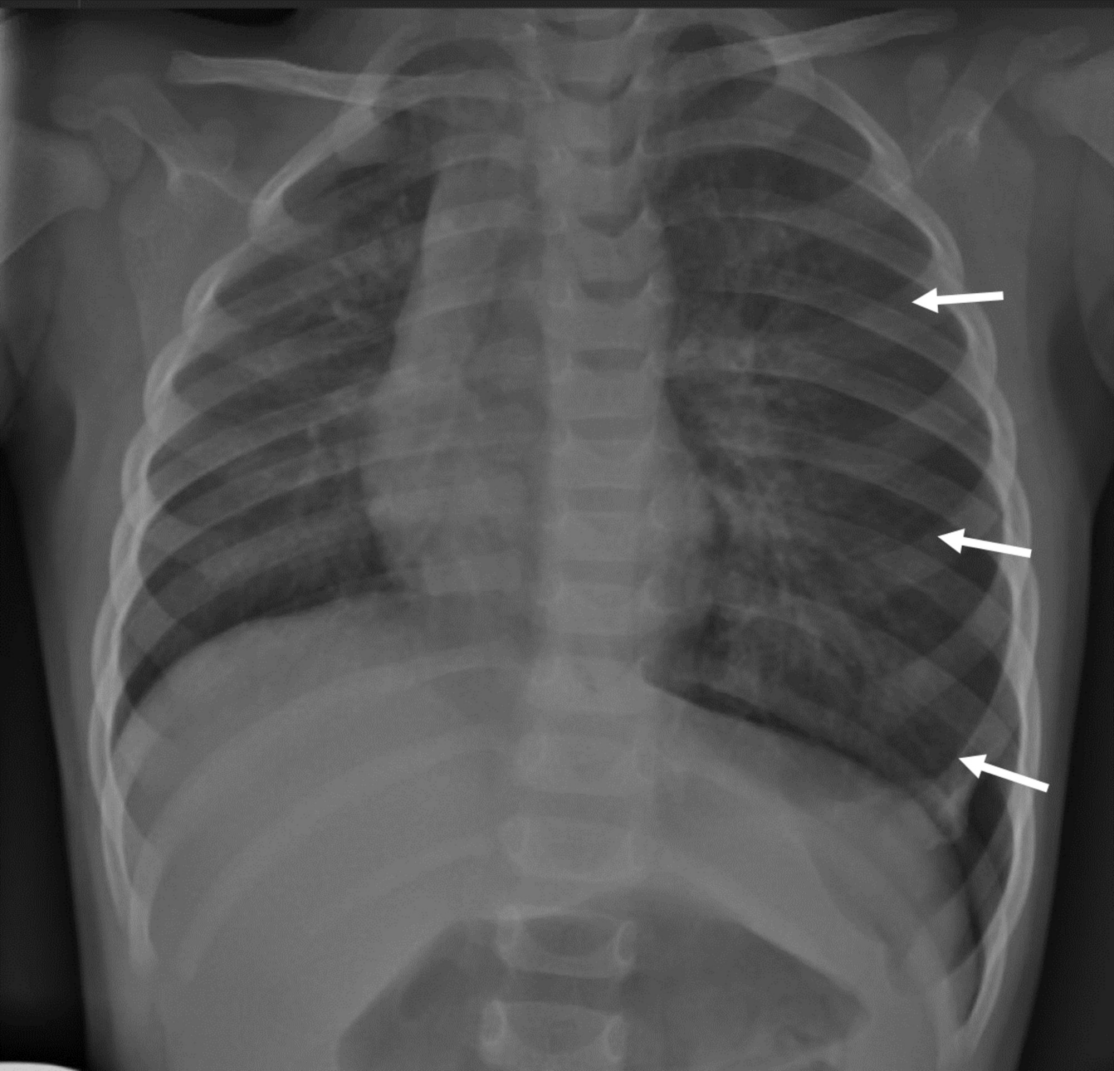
Chest X-ray on day five demonstrated increased left-sided pneumothorax with a mediastinal shift, despite the patient’s clinical improvement; white arrows outline the lateral aspect of the lung
